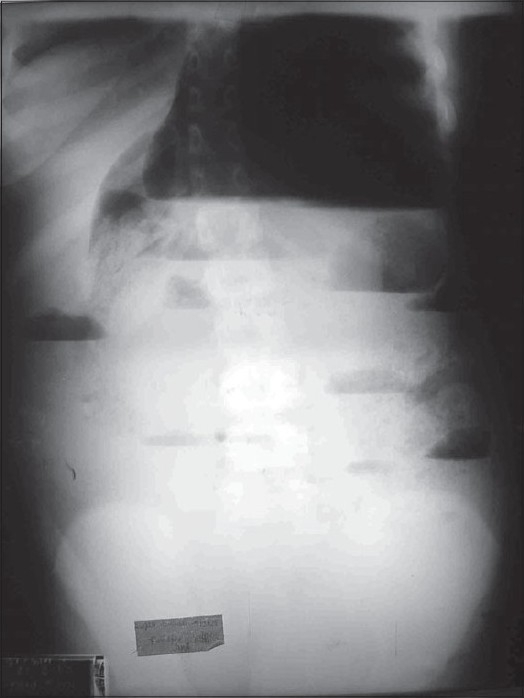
Plain X-ray Abdomen Showing Hugely Dilated Shadow
